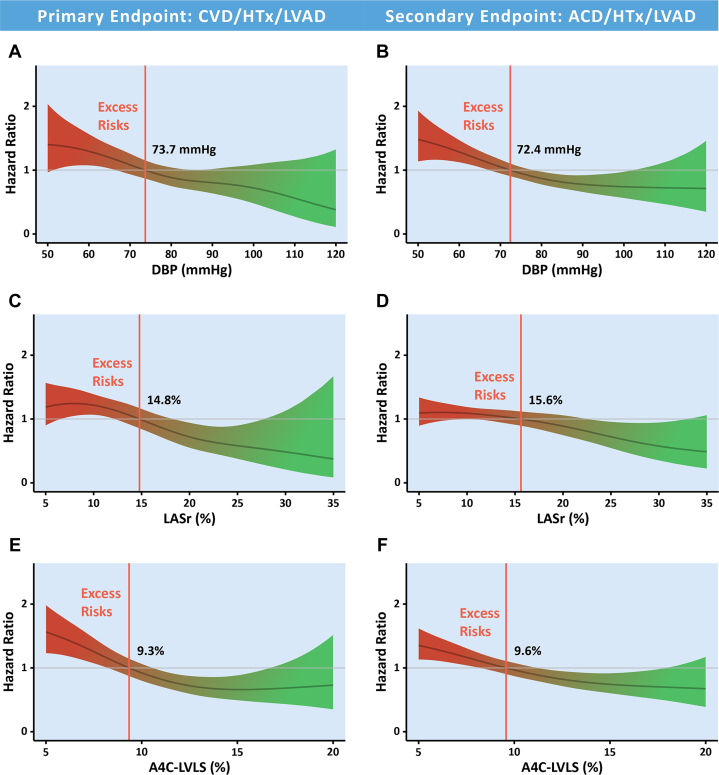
Adjusted Splines for Primary and Secondary Endpoints The HR = 1 line indicates the cohort's average mortality, with excess risk present at values >1. Spline curves adjusted for stratified age (cutoff at 70 years), sex, right ventricular fractional area change (RV-FAC), and procedures revealed increased risk of the primary endpoint (cardiovascular death [CVD]/heart transplantation [HTx]/left ventricular assist device [LVAD]) and secondary endpoint (all-cause death [ACD]/HTx/LVAD) when the diastolic blood pressure (DBP) was (A) <73.7 mm Hg and (B) <72.4 mm Hg, respectively. (C, D) Increased risk of primary and secondary endpoints when left atrial reservoir strain (LASr) was <14.8% and <15.6%, respectively. Increased risk of primary and secondary endpoints when left ventricular longitudinal strain from the apical 4-chamber view (A4C-LVLS) was (E) <9.3% and (F) <9.6%, respectively. Red-shaded areas indicate excess risk, while green-shaded areas represent protective ranges associated with lower risk. Vertical dashed lines mark risk inflection thresholds.
